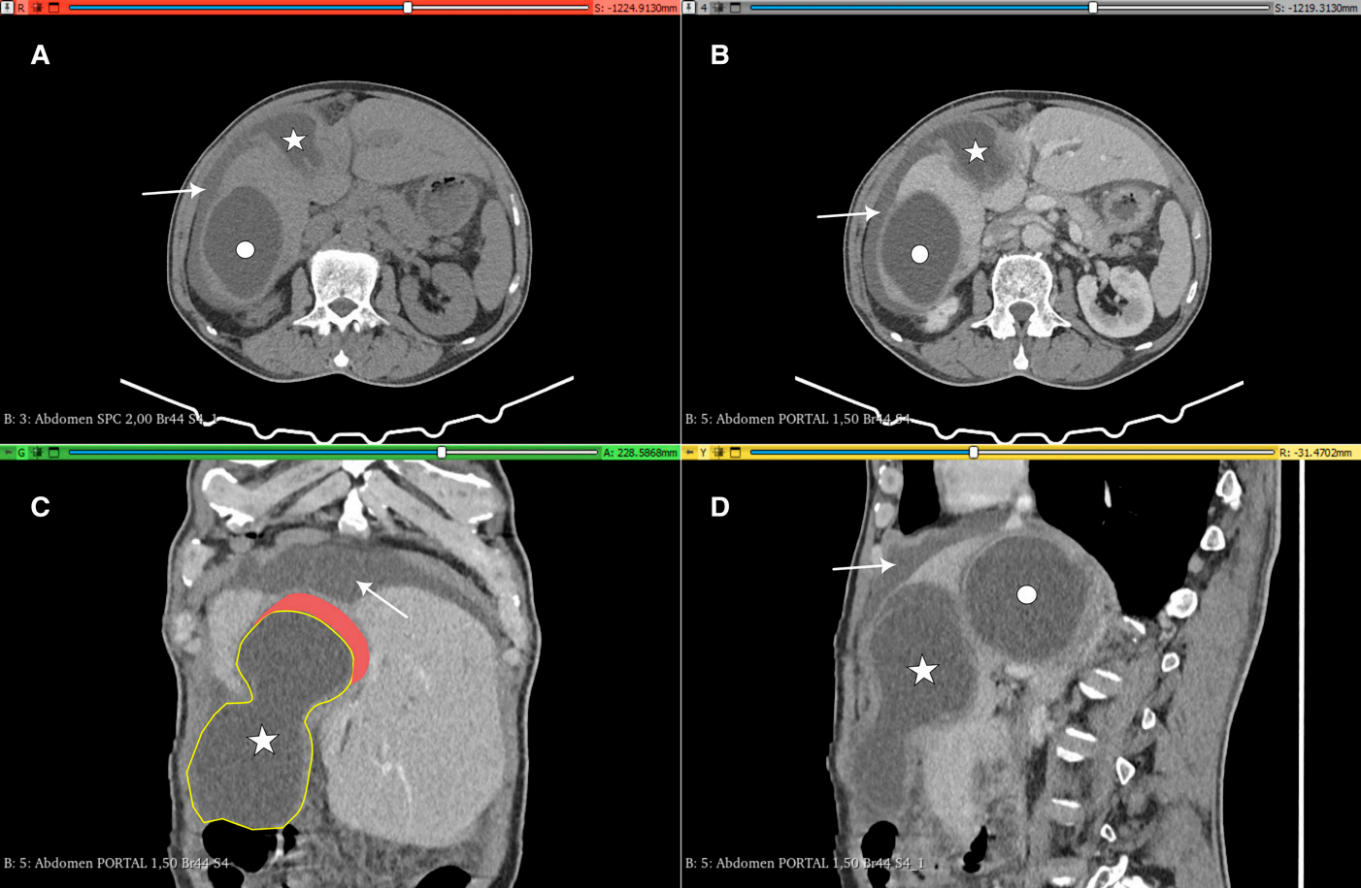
Preoperative computed tomography scan. (A) Nonenhanced axial view, (B) portal phase axial view, (C) portal phase coronal view, and (D) portal phase sagittal view, showing two hydatid cysts in the liver (white circle and white star). Perihepatic effusion is indicated by white arrows. The hourglass shape is depicted by a yellow contour in panel C. The cleavage plane between the laminated membrane and the pericyst is highlighted in red in Panel C.
